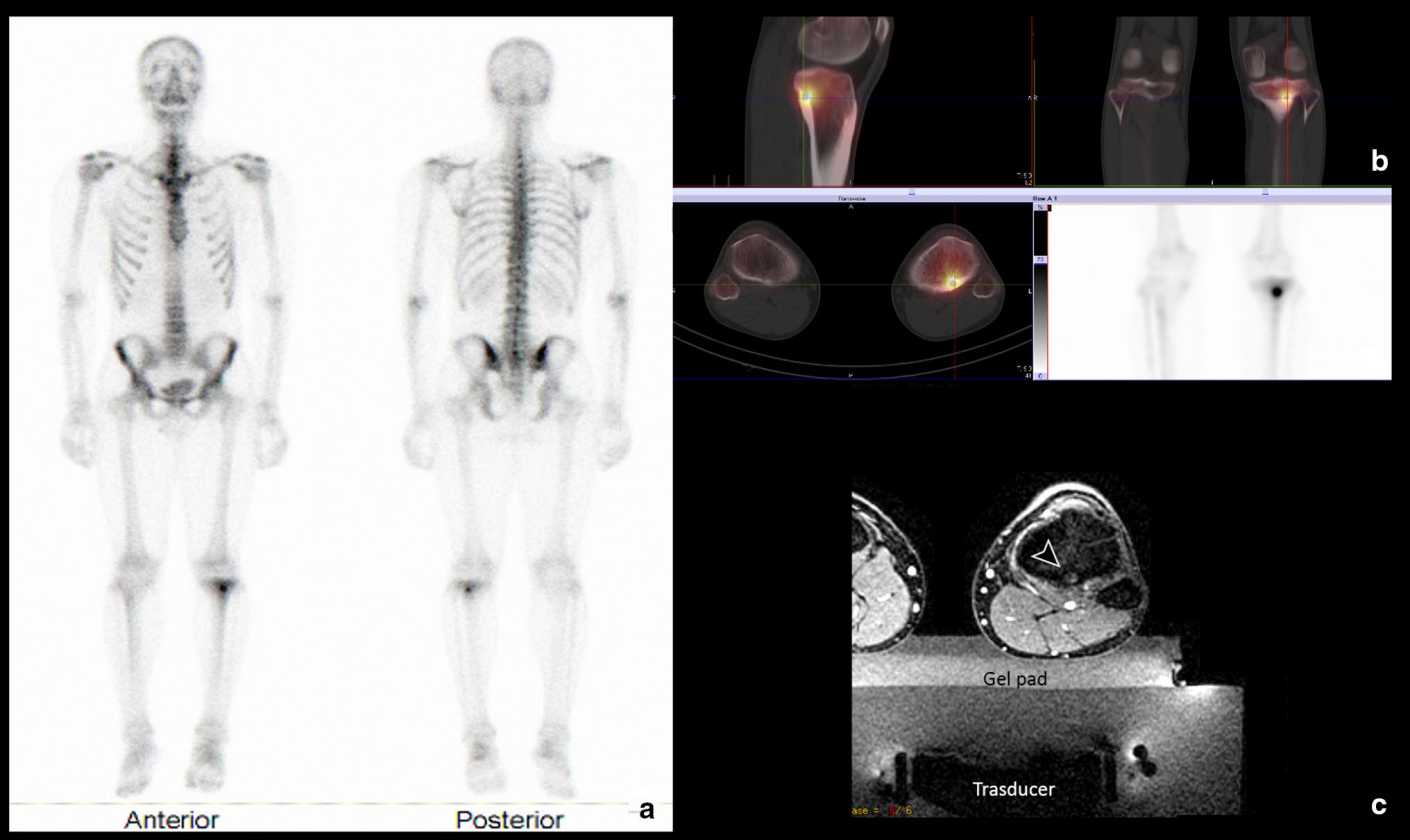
Osteoid osteoma of the left proximal tibial meta-diaphysis. a Technetium-99 m bone scintigraphy shows the typical double density sign, characterized by a central focus of intense radiotracer uptake (the nidus) within a region of generalized increase activity. b SPECT/CT multiplanar images demonstrate increased radiotracer uptake at the site of the nidus. c Axial MRI T2-weighted image with fat saturation during MRg-FUS shows treatment planning and osteoid osteoma location (arrowhead) relative to the transducer
